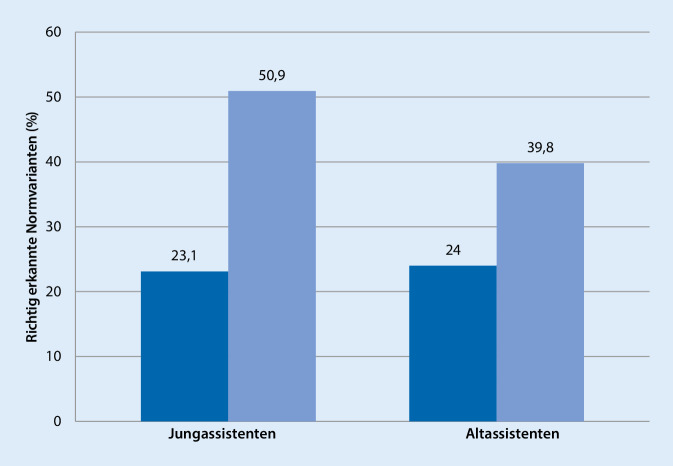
Die Ergebnisse der subjektiven Evaluation sind in Tab. 2 zusammengefasst. 91,7 % der Assistentzärzt*innen fanden die CLOSE-Kriterien sinnvoll, und 83,3 % gaben an, dass sie sicherstellen, dass sie sich genug Zeit für die Beurteilung der CT-Bilder nehmen. 75 % der Assistent*innen stimmten vollkommen zu, und 25 % stimmten eingeschränkt zu, dass sie die Kriterien sicherer im Umgang mit der Anatomie und CT-Bildern der Nasennebenhöhlen machen. 83,3 % stimmten nicht zu, dass die CLOSE-Kriterien überflüssig seien. Alle Teilnehmer*innen stimmten vollkommen zu, dass die präoperative Checkliste ein sinnvolles Instrument ist, um chirurgische Gefahrenpunkte zu identifizieren und die Sicherheit der Operation zu erhöhen. Alle Teilnehmer*innen gaben, dass sie die Kriterien weiterhin verwenden werden. Die Tab. 2 zeigt die Ergebnisse der Evaluation mit Mittelwert und Standardabweichung.
